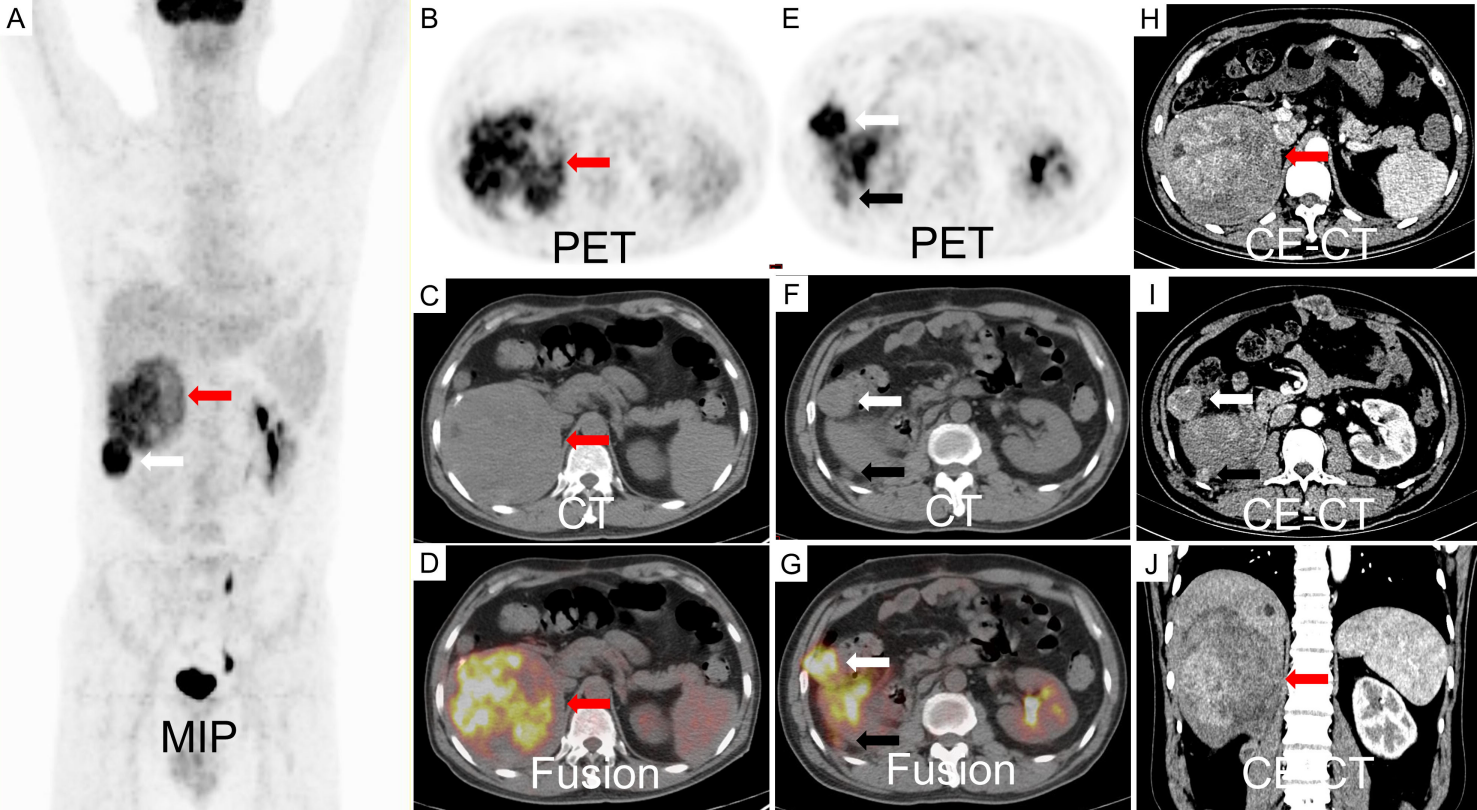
An ^18^F-FDG PET/CT scan revealed a large, inhomogeneous hypermetabolic mass in the liver, characterized by a SUVmax of 8.8, as indicated by the red arrow. Additionally, two hypermetabolic metastases were identified adjacent to the liver capsule, with SUVmax values of 12.1 (white arrow) and 3.1 (black arrow), respectively (A-G). The contrast-enhanced CT scan demonstrated inhomogeneous enhancement of the metastases during the arterial phase (H-J).
